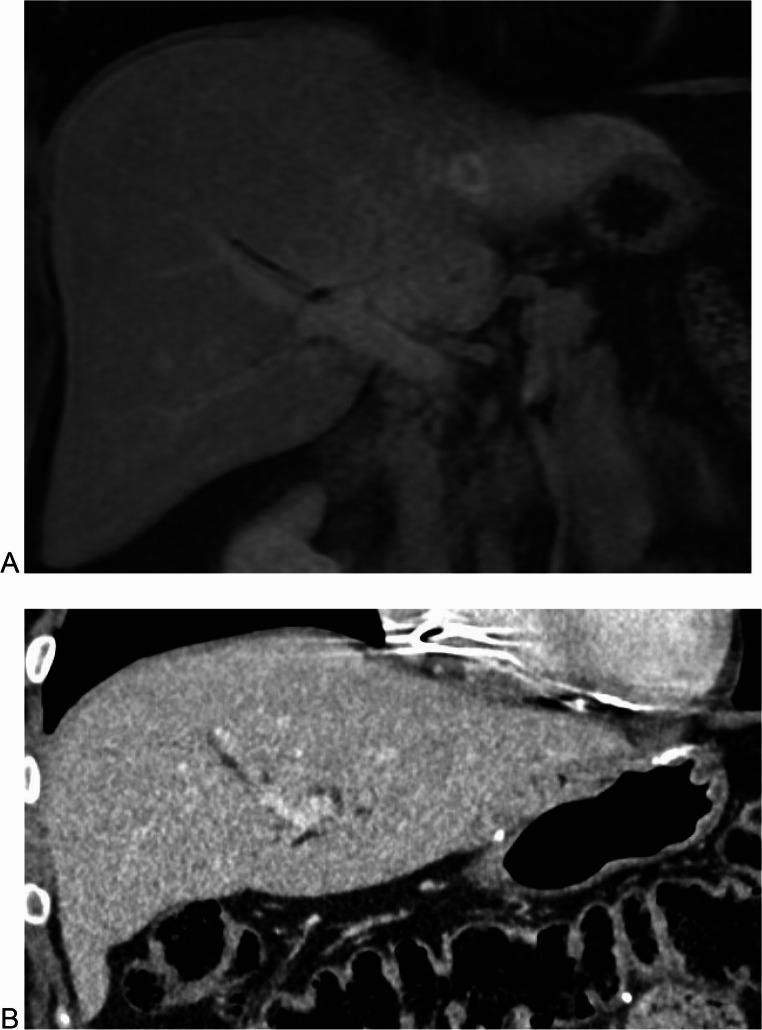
Representative cross-sectional images from a patient with HA pre- and post-bariatric surgery (Roux-en-Y gastric bypass). (A) MRI showing hepatic adenoma in segment II (1.8 cm x 1.4 cm) (B) CT captured during post-operative follow-up without evidence of HA in segment II. Patient’s BMI pre-op was 38.03 kg/m^2^ and at time of follow-up imaging was 23.73 kg/m^2^
